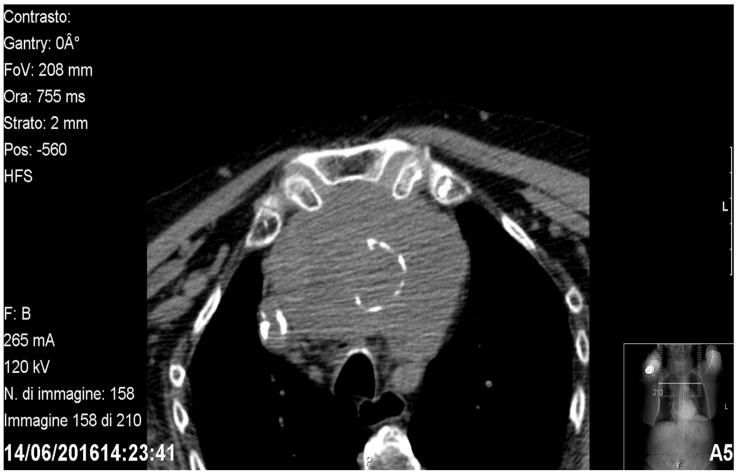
Axial CT showing a prevascular retrosternal goitre.
